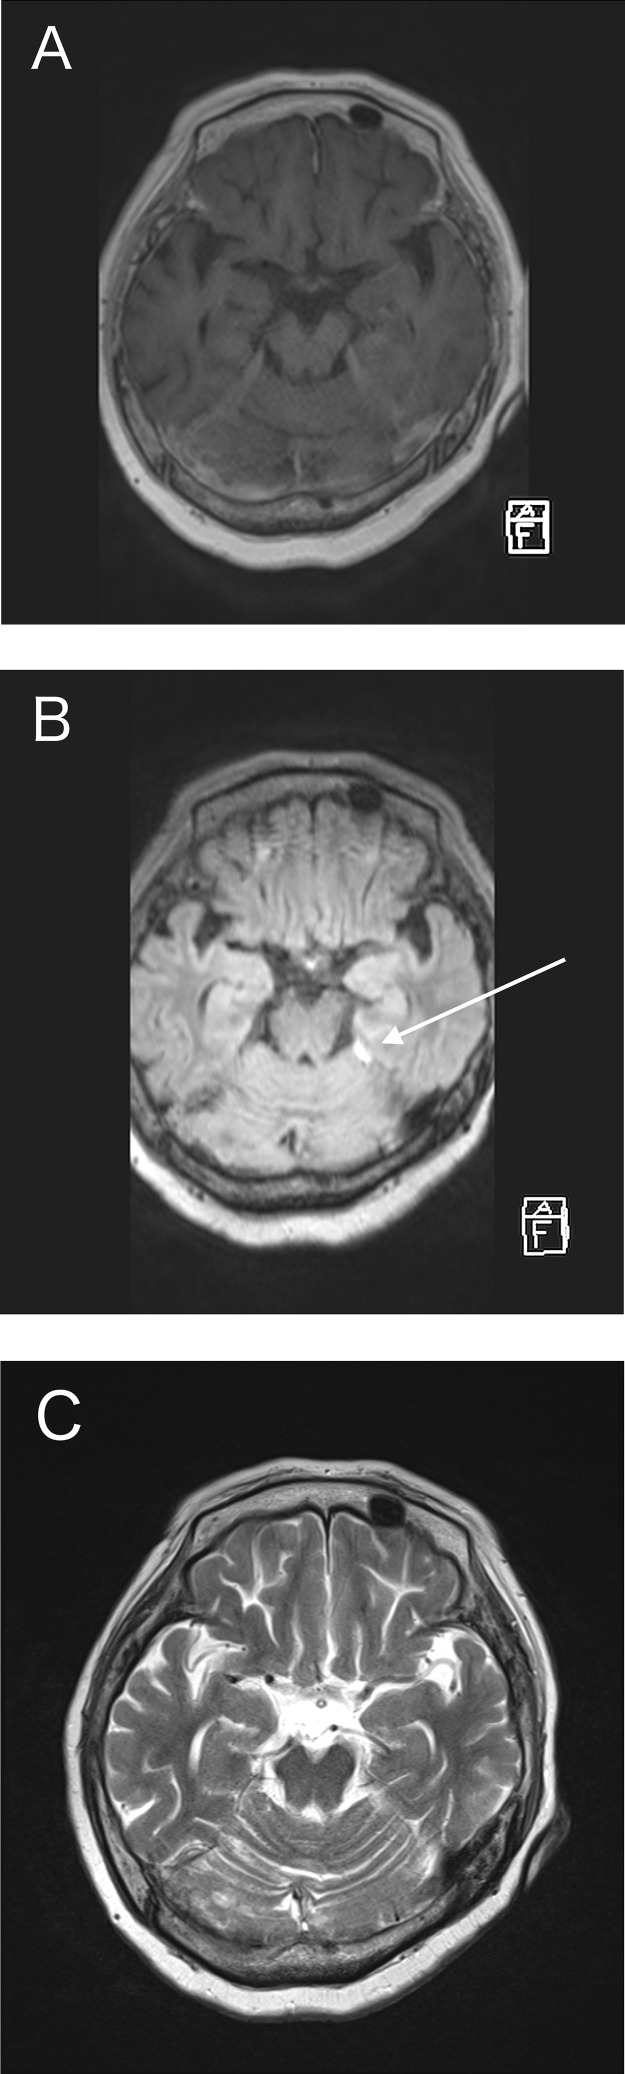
(A) CE-T1WI could not depict a thickened tentorium. (B) CE-FLAIR virtually demonstrated the thickened tentorium on the left side (arrow). (C) The tentorium was not detected on T2WI.
